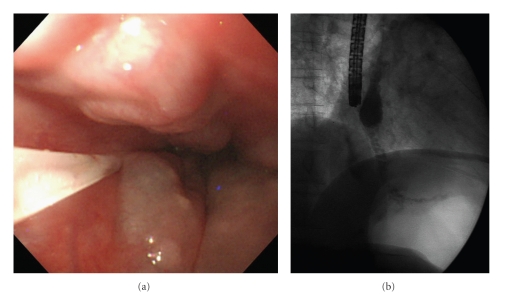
EIS procedure. (a) EIS was performed by intravariceal injection of 15 mL of 5% EOI in total. (b) Radiographic imaging showed that 5% EOI reached the cardiac plexus and LGV.
